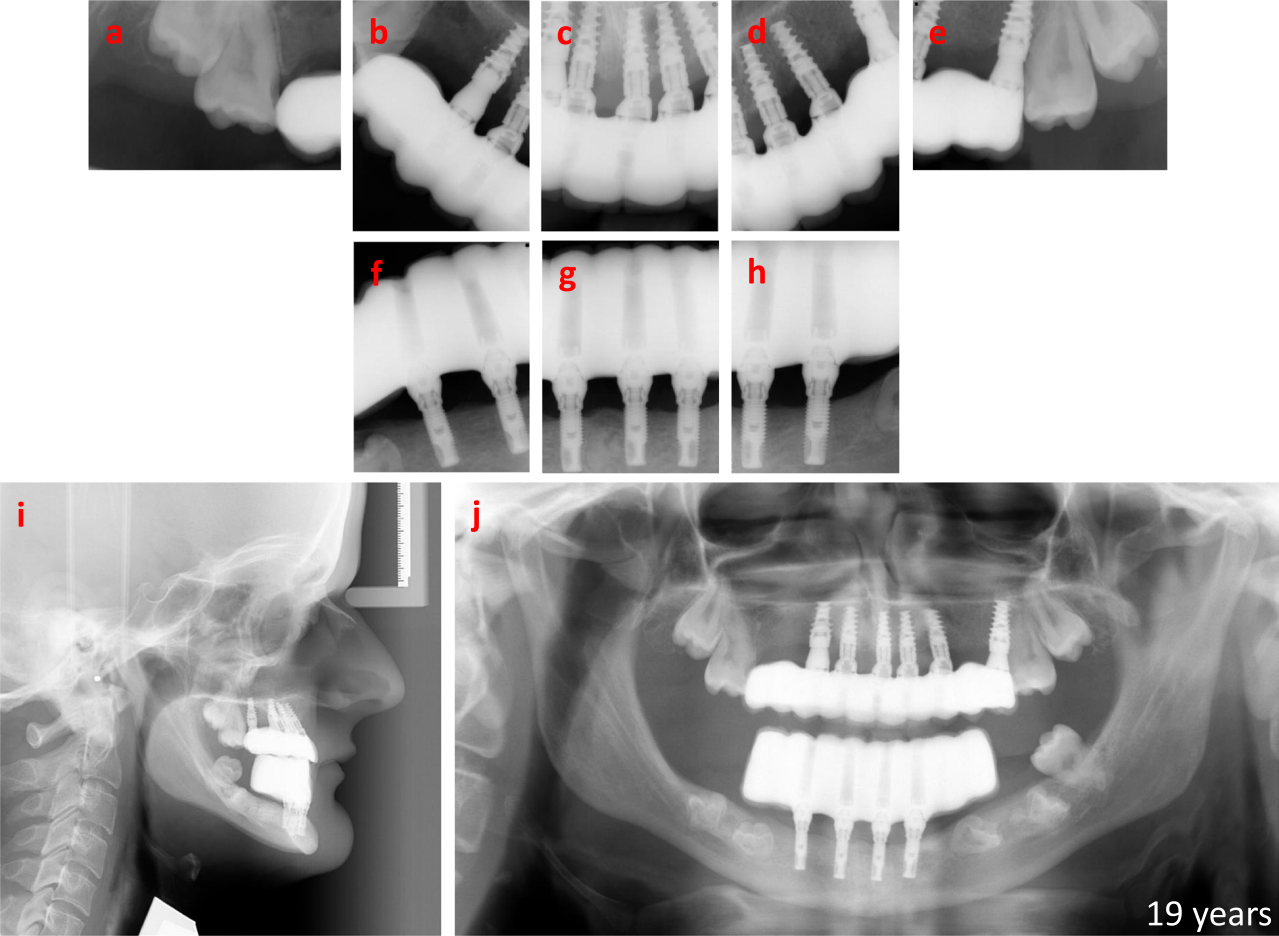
Radiographic overviews of the implant-fixed bridges at the time of initial loading at the age of 19 years. Radiographs of the screw retained-implant bridges with multi unit abutments at the stage of initial loading: a-e intraoral radiographs of the upper jaw, f–h intraoral radiographs of the lower jaw, i radiographic sagittal view, and j radiographic panoramic view
